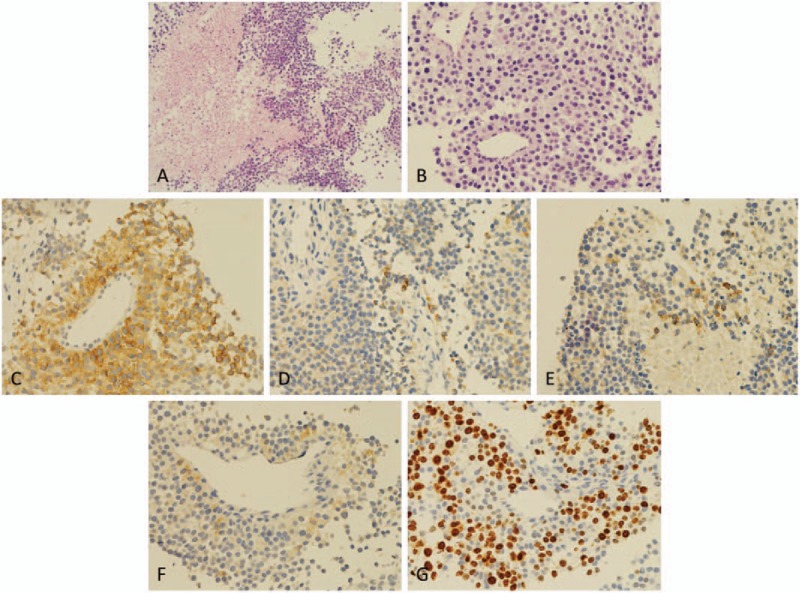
(A and B) Posteroanterior (PA) and lateral x-ray image of the lumbar spine obtained postoperatively.
